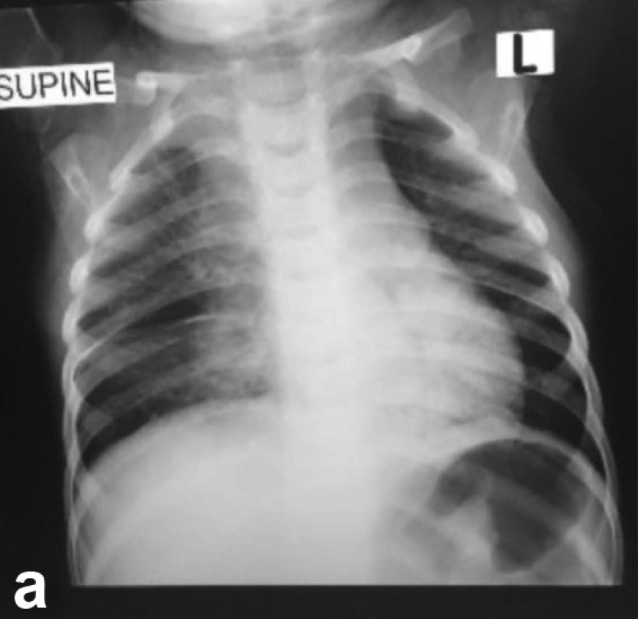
Chest X-ray on diagnosis of unconfirmed tuberculosis at the local
